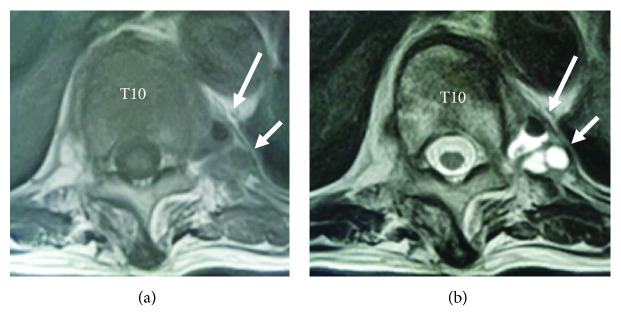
Preoperative axial T1-weighted (a) and T2-weighted (b) magnetic resonance imaging, showing the gas (long arrows pointing T1-low and T2-low signal intensity areas) and fluid (short arrows pointing T1-iso and T2-high signal intensity areas) collections just below the left 10th rib head.
